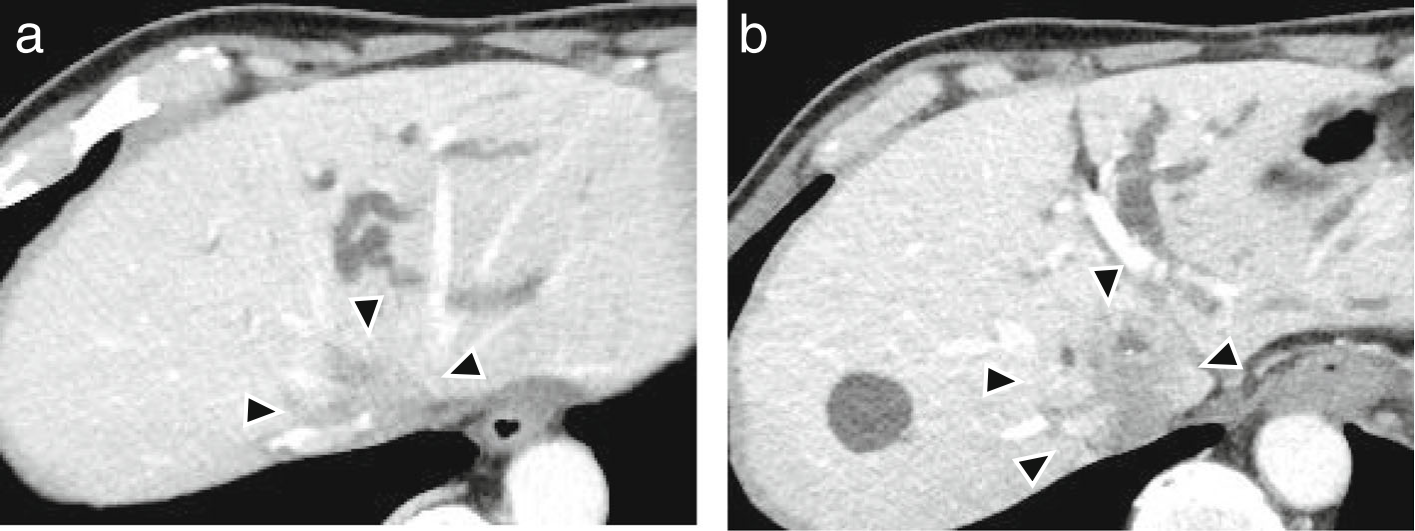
a Computed tomography showed an irregular tumor (arrowheads) involving the inferior vena cava and the root of the three major hepatic veins. b Left hepatic bile duct was dilated due to tumor invasion
